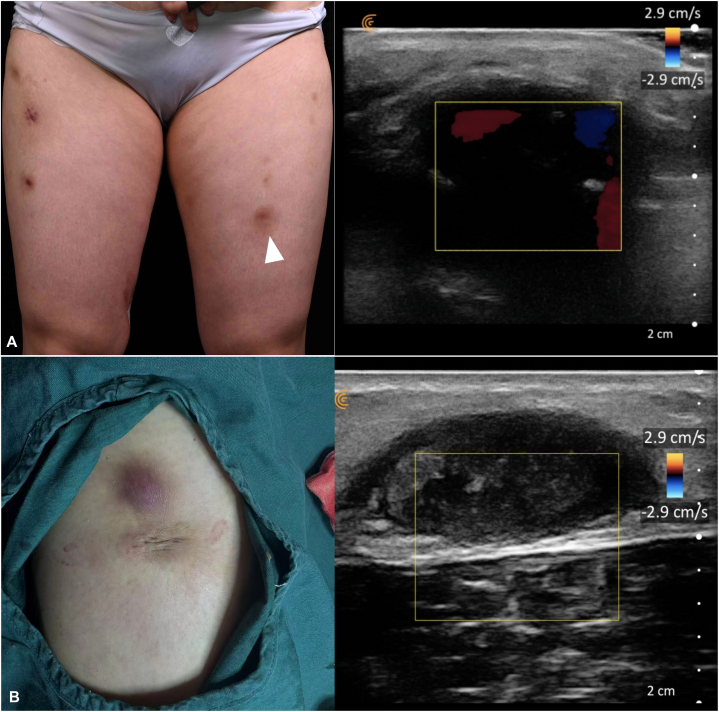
Doppler ultrasonography of the left thigh lesion. A, Prominent intralesional vascular signals after treatment interruption (*white triangle*). B, Disappearance of vascular signals after 4 months of continuous therapy.
